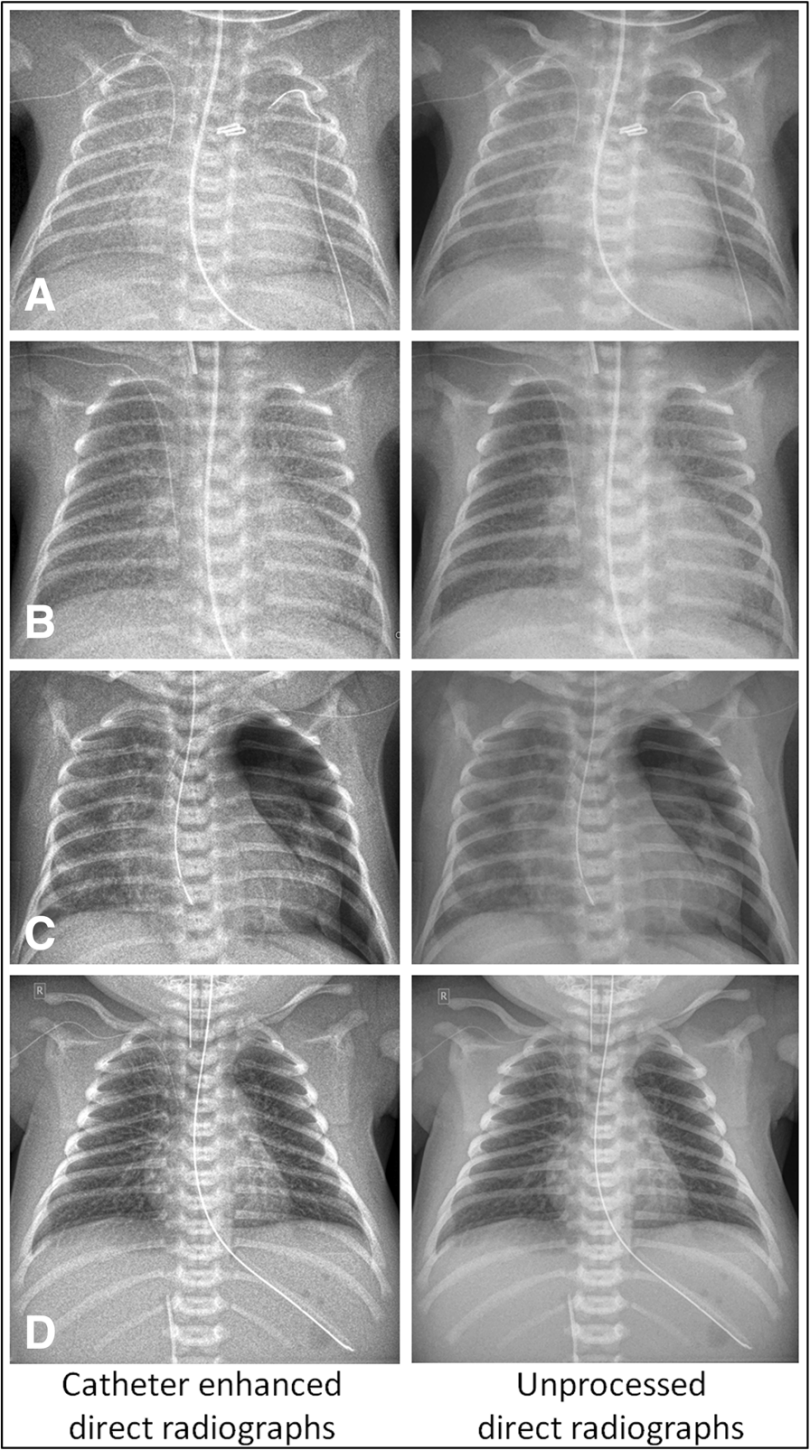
Exemplary comparisons of catheter-enhanced and unprocessed direct radiographs. a A central catheter is inserted in a vein of the right upper limb and ends in the superior vena cava. Main diagnosis: Left-to-right shunting with pulmonary hypervolemia, shortly after surgical ligation of a patent ductus arteriosus (PDA). b A central catheter is inserted in a vein of the right upper limb and ends in the right atrium. Main diagnosis: Infant respiratory distress syndrome (IRDS). c A central catheter is inserted in a vein of the left upper limb and ends in the superior vena cava. Main diagnosis: Two-sided pneumonia, left-sided pneumothorax. d A central catheter is inserted in a vein of the right upper limb and ends in the superior vena cava. Main diagnosis: Transient tachypnea of the newborn (TTN)
